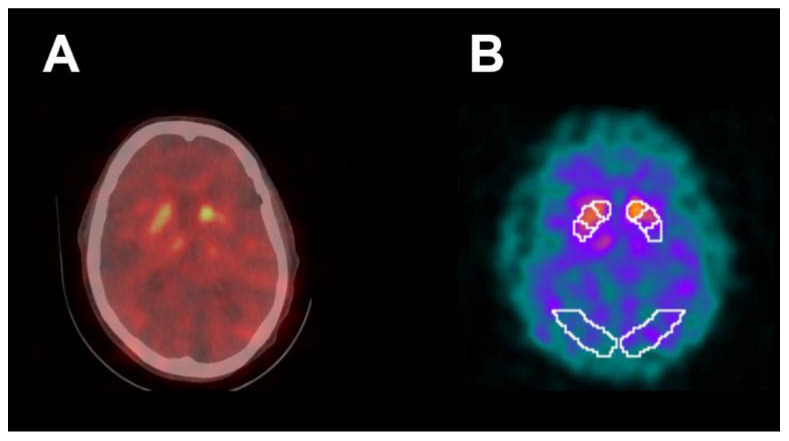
Abnormal [^123^I]FP-CIT SPECT/CT (A) in a 76-year-old right-handed male demonstrating decreased activity in the striatum bilaterally, particularly in the left putamen. [^123^I]FP-CIT SPECT images (B) co-registered to the DaTQUANT software template demonstrated a left putamen SBR of 0.6 with z-score of −3.93. UPDRS-III motor score was 5 and MoCA score was 20. Patient was not receiving medication for PD. For contralateral right upper extremity, VR time was 50 s, VR ADL Score was 9.8, VR Rest Tremor Power was 1.48 × 10^−8^ m^2^/Hz, and VR Posture Tremor Power was 6.09 × 10^−8^ m^2^/Hz.
